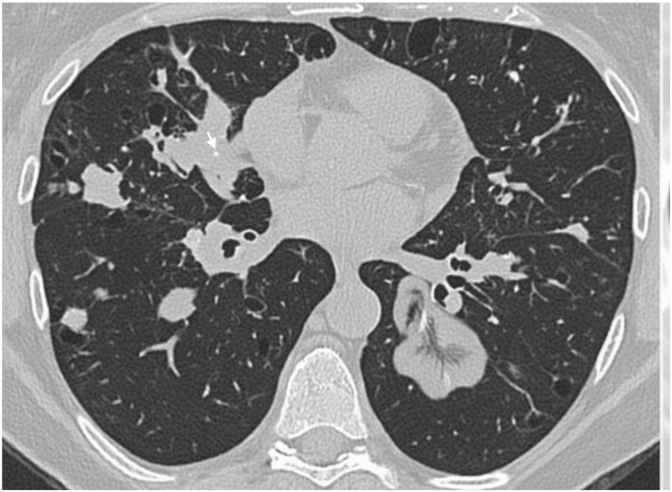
CT Chest shows multiple bilateral lung nodules, some with central calcifications (arrow).
